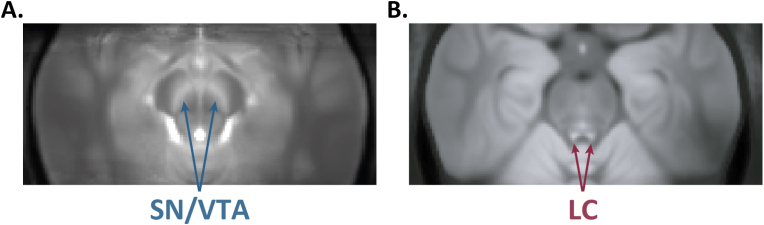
Two examples of NM-MRI images. The images depict the average contrast-to-noise ratio across several individuals in MNI standard space for (A) the substantia nigra/ventral tegmental area (SN/VTA), acquired using a gradient-recalled echo (GRE) sequence with magnetization transfer pulses; and (B) the locus coeruleus (LC), acquired using a GRE sequence with dual-slab saturation pulses.
